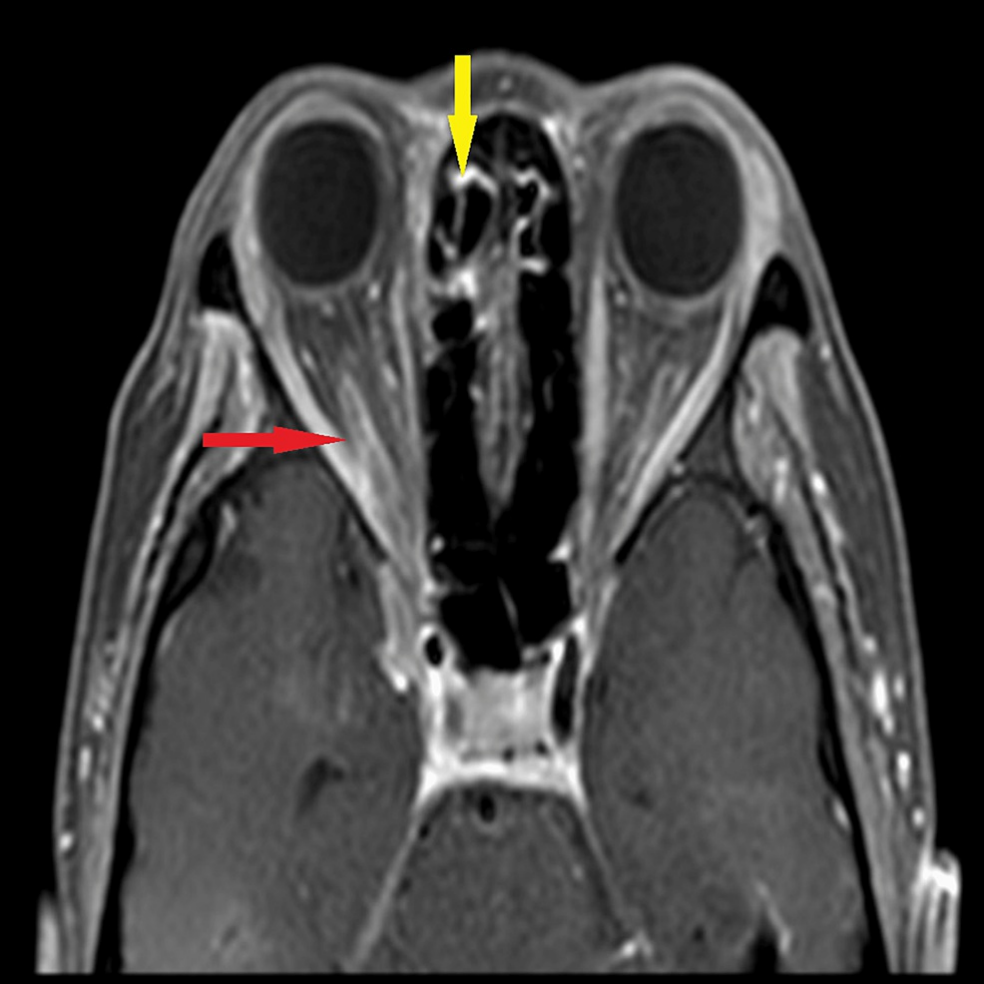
Axial cut of contrast MRI of the brain and orbit showing enhancement of the right optic nerve sheath (red arrow) and mucosal enhancement of the right ethmoid sinus (yellow arrow) MRI: magnetic resonance imaging
